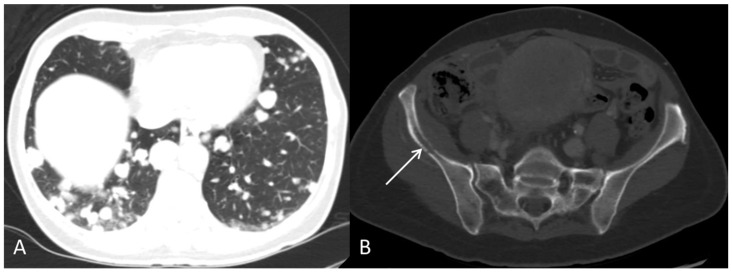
The contrast-enhanced CT, after approximately two months of sorafenib treatment, showed progression with multiple pulmonary metastases (A) and an osteolytic metastasis with corticalis destruction in the right pelvic bone ((B), arrow). S-AFP was 412,200 kIU/L. Sorafenib was discontinued due to progression. The patient passed away shortly after.
